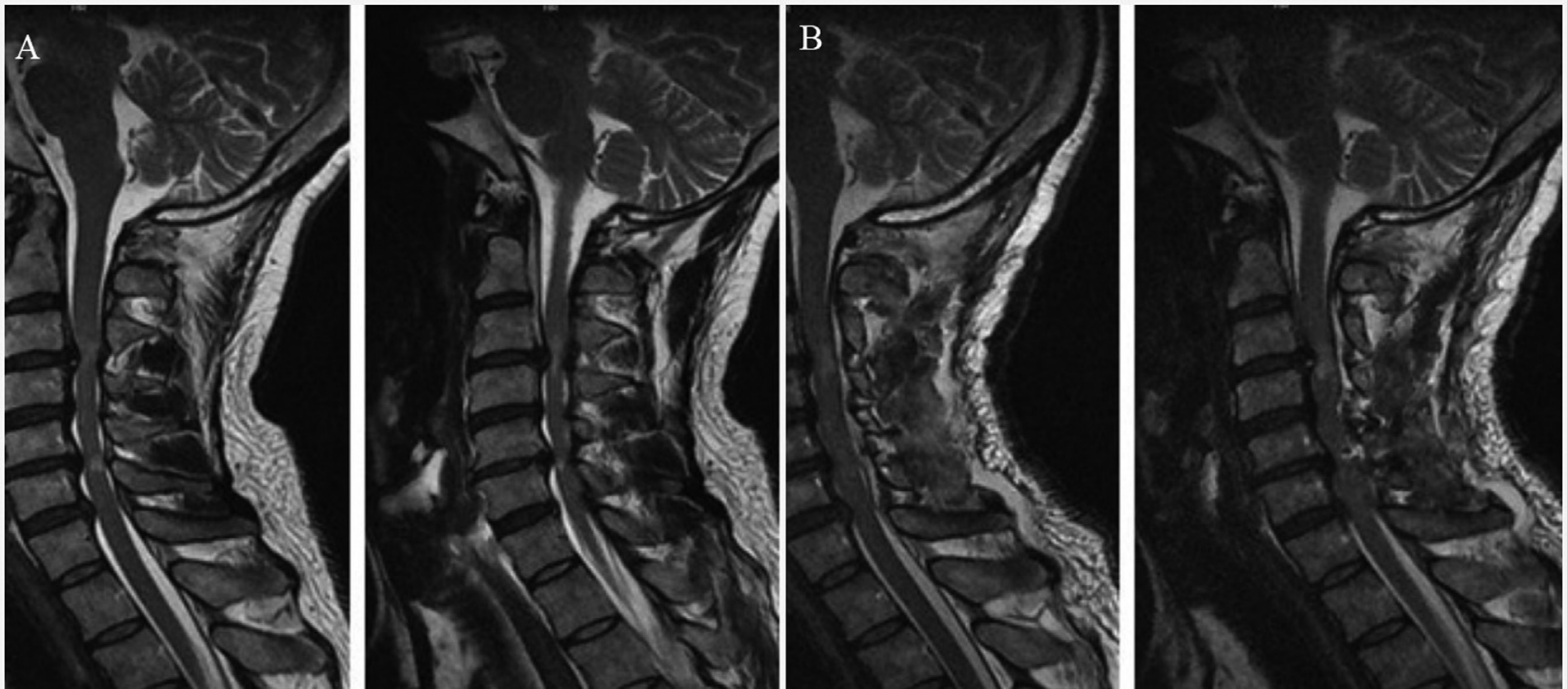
Cervical spinal cord T2-weighted MRI revealing severe degenerative disk disease and compressive spondylotic myelopathy at the levels of C3-C4 and C5-C6 (
